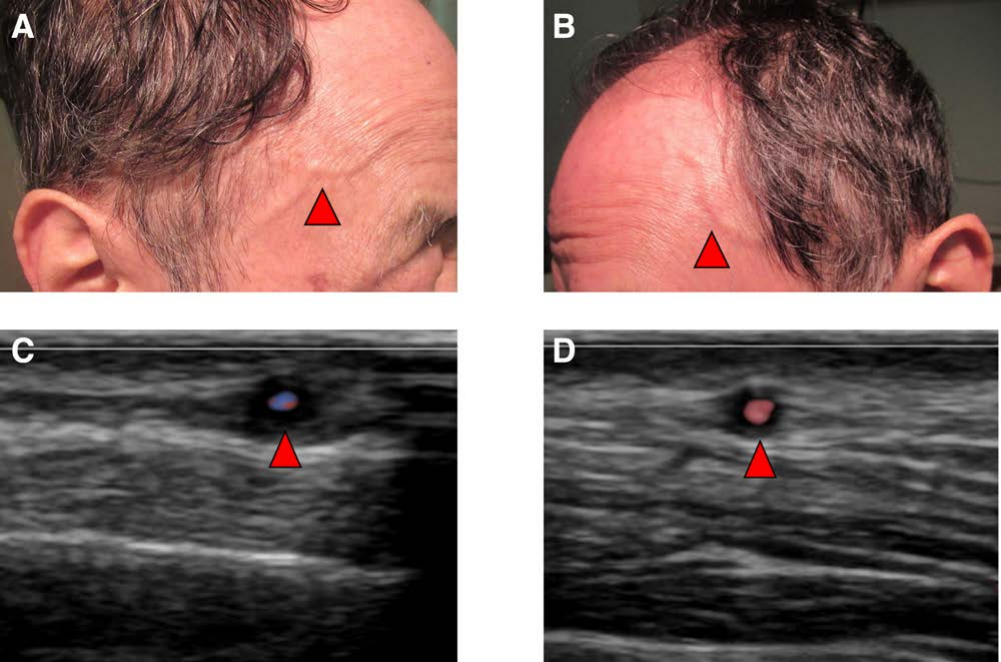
Bilateral temporal arteries of the patient. Nodular swelling of the right temporal artery (A, arrows) and left temporal artery (B, arrows) was observed. Ultrasound echography showing a halo sign surrounding both the right (C, arrows) and left (D, arrows) temporal arteries.
